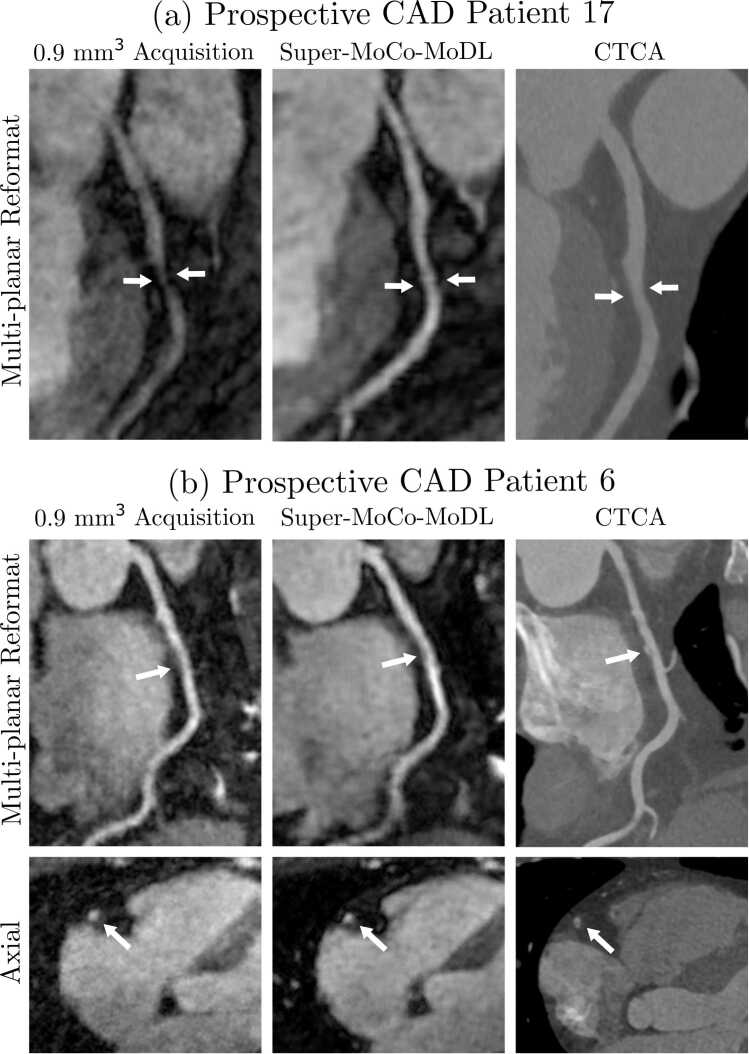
Comparison of NR-PROST reconstructions of high-resolution CMR acquisitions, the proposed Super-MoCo-MoDL approach as applied to prospective low-resolution CMR, and CTCA. (a) Multi-planar reformats of the LAD demonstrating CAD causing stenosis in the mid vessel (white arrows). The lesion appears more severe on the 0.9-mm isotropic CMRA compared to both the Super-MoCo-MoDL CMRA and the CTCA. Vessel wall contrast and delineation are improved with the Super-MoCo-MoDL reconstruction. (b) Multi-planar reformats (top) and axial views (bottom) of the RCA in a patient with mixed plaque in the mid vessel. White arrows indicate the location of the partially calcified plaque. *CAD* coronary artery disease, *CMR* cardiac magnetic resonance, *CMRA* coronary magnetic resonance angiography, *CTCA* computed tomography coronary angiography, *LAD* left anterior descending artery, *NR-PROST* non-rigid motion-corrected patch-based low-rank reconstruction method, *RCA* right coronary artery
